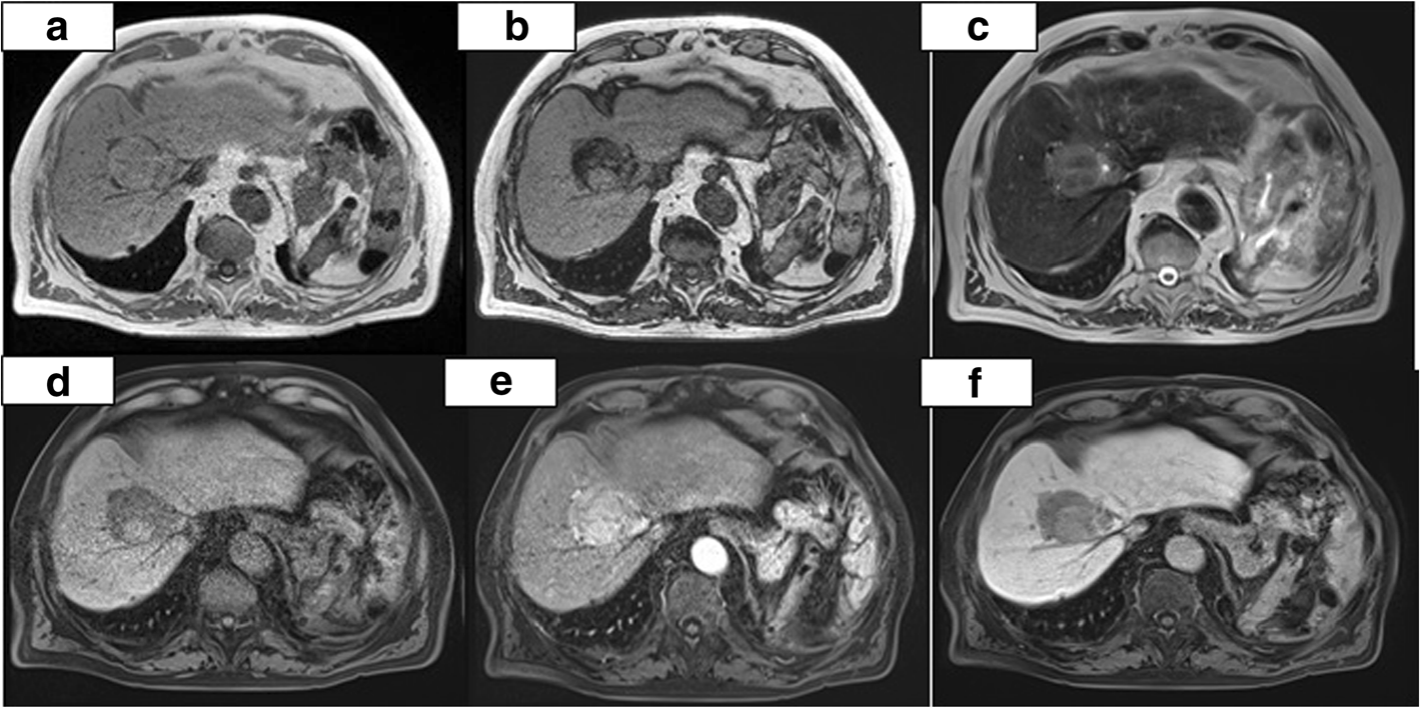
a–f Abdominal MRI, December 4, 2014. T1 on phase (a) and T1 out of phase (b) images confirm the increase in volume of the nodule that shows abundant fatty component inside. On T2 (c), the nodule shows heterogeneous hyperintensity. The dynamic study (d) shows the hypervascularization and the rapid washout of the nodule. In the hepatobiliary phase (f), the nodule appears hypodense with two components that reflect the heterogeneity of the lesion. Note the well-defined borders. All these characteristics allow to define the nodule as suspected HCC
